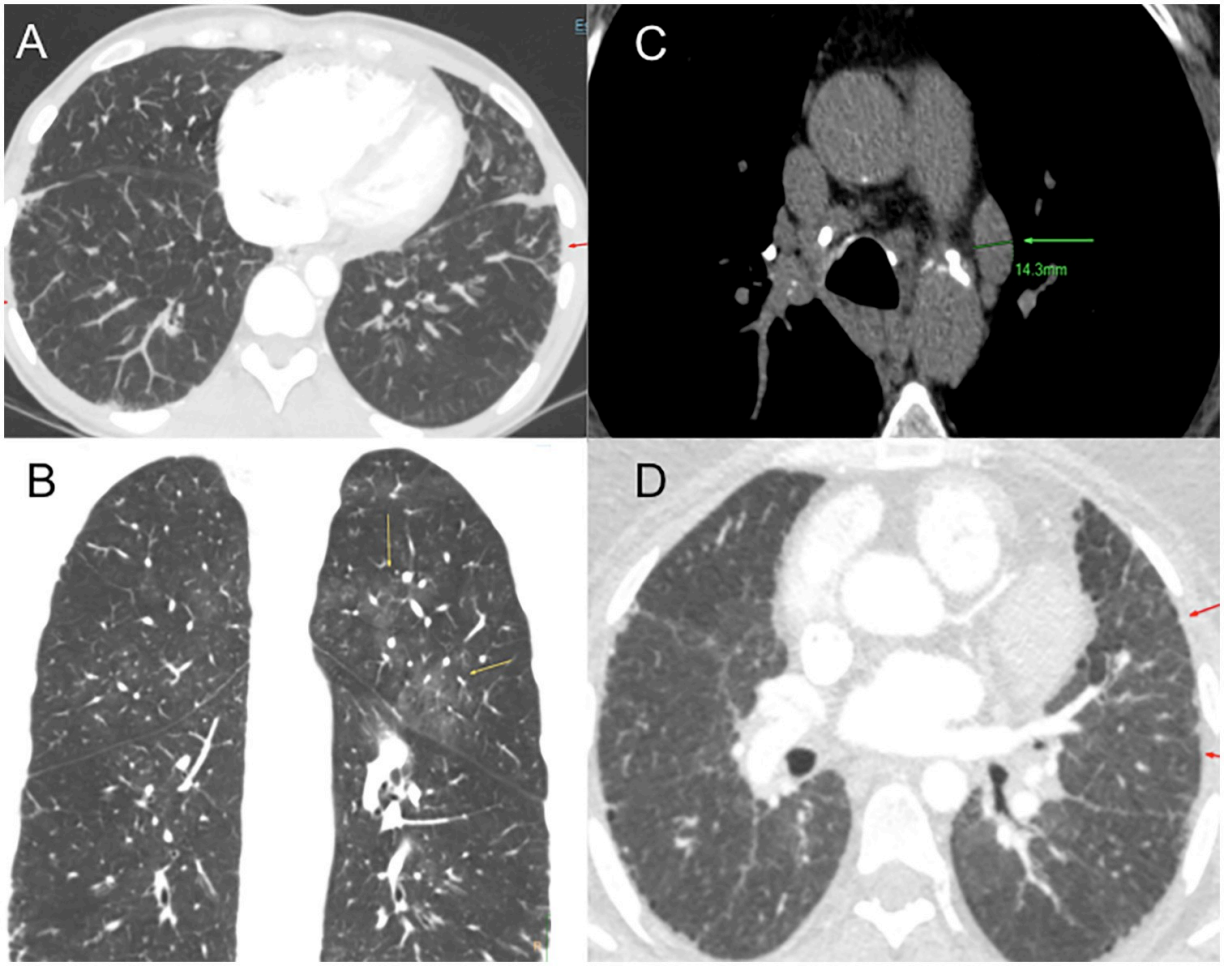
Multidetector Computed Tomography patients 3 and 4. (A) Patient 3 interlobular septal thickening (red arrow) (B) Patient 3 ground grass pattern (yellow arrow) (C) Patient 4 mediastinal lymphadenopathies (green arrow) (D) Patient 4 interlobular septal thickening (red arrows).
